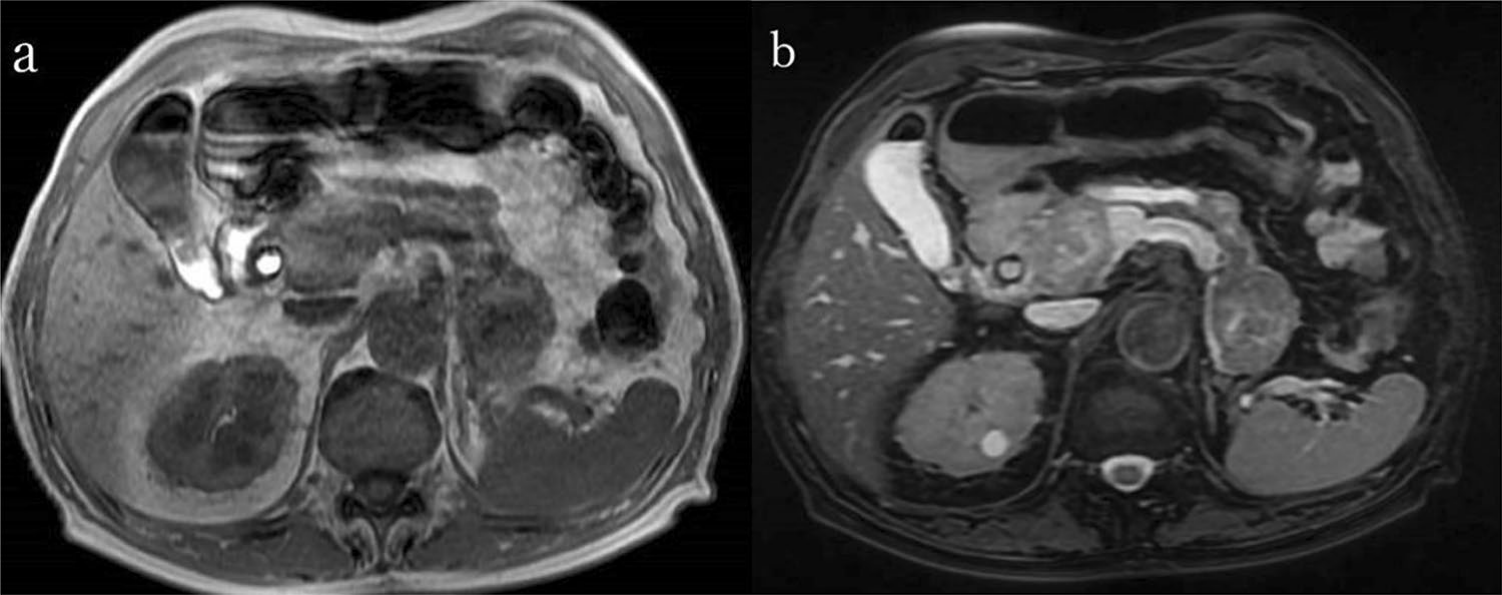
a Magnetic resonance imaging showed multiple tumors in the pancreas with heterogeneously low signal intensity on the T1-weighted image. Bile in the common bile duct and gallbladder neck has high intensity, which suggests hemobilia. b Pancreatic masses have rather low intensity on T2WI, suggesting intratumor bleeding. Area with high intensity in pancreas head mass is considered as tumor necrosis
